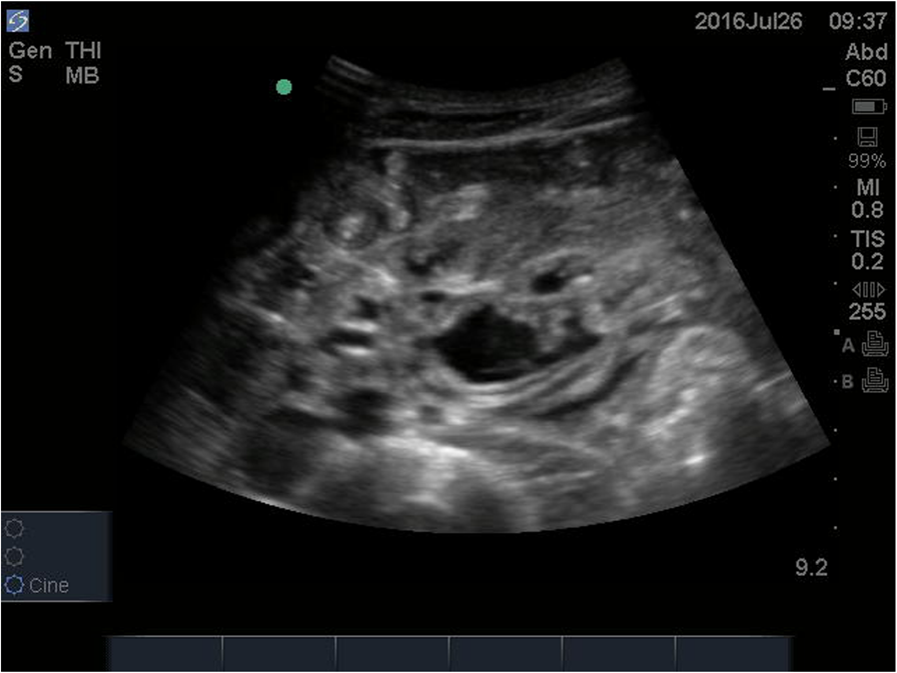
Abdominal ultrasound scan
